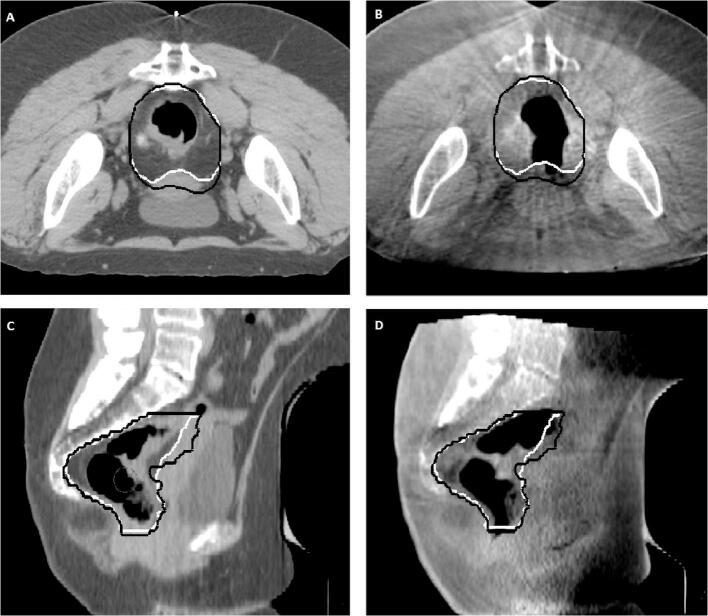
Delineation of the mesorectal part of the CTV on pCT (A, C) and CBCT (B, D) for a female patient. Both transversal (A, B) and sagittal view (C, D) are shown. White and black lines indicate the target delineation according to pCT and CBCT, respectively.
